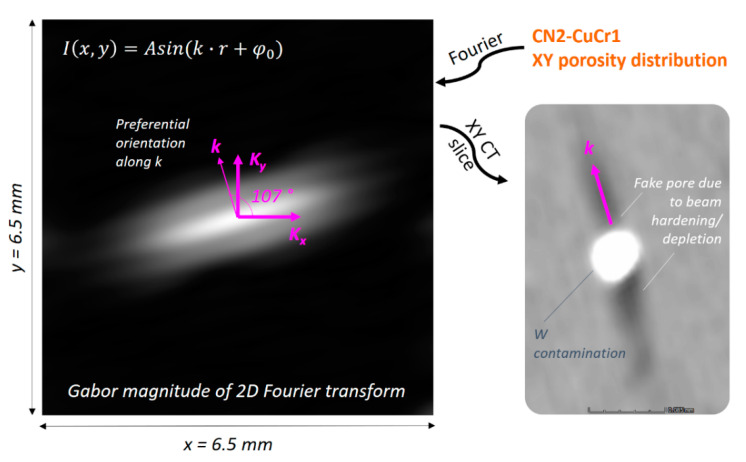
Gabor magnitude of the 2D Fourier transform for the CN2-CuCr1 CT dataset (XY plane), to highlight preferential spatial frequencies; the repeating pattern oriented at 107° is identified as a streaking artefact generated by the W contaminants due to X-ray beam hardening and partial beam depletion.
